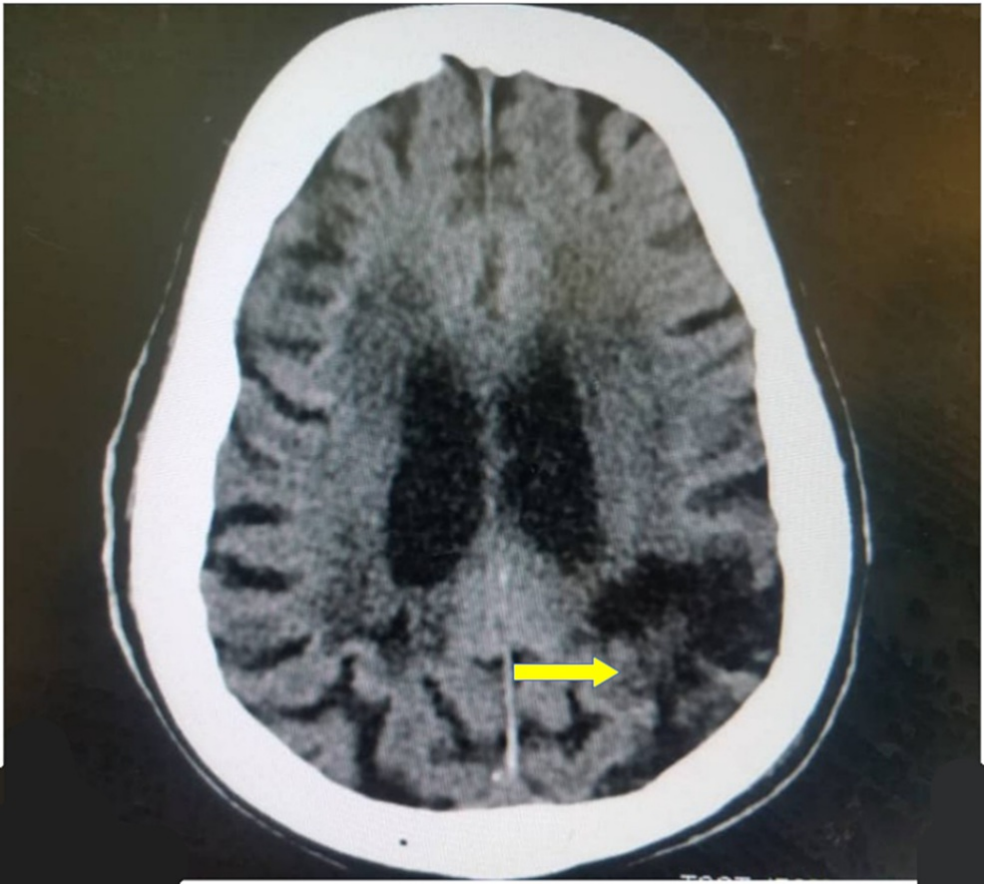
An axial non-enhanced CT scan of the brain of a 62-year-old female without a history of hypertension, who presented with a sudden onset of right-sided hemiplegia, showing a hypodense area at the left parieto-occipital region (yellow arrow) in keeping with intracerebral infarct (chronic).
